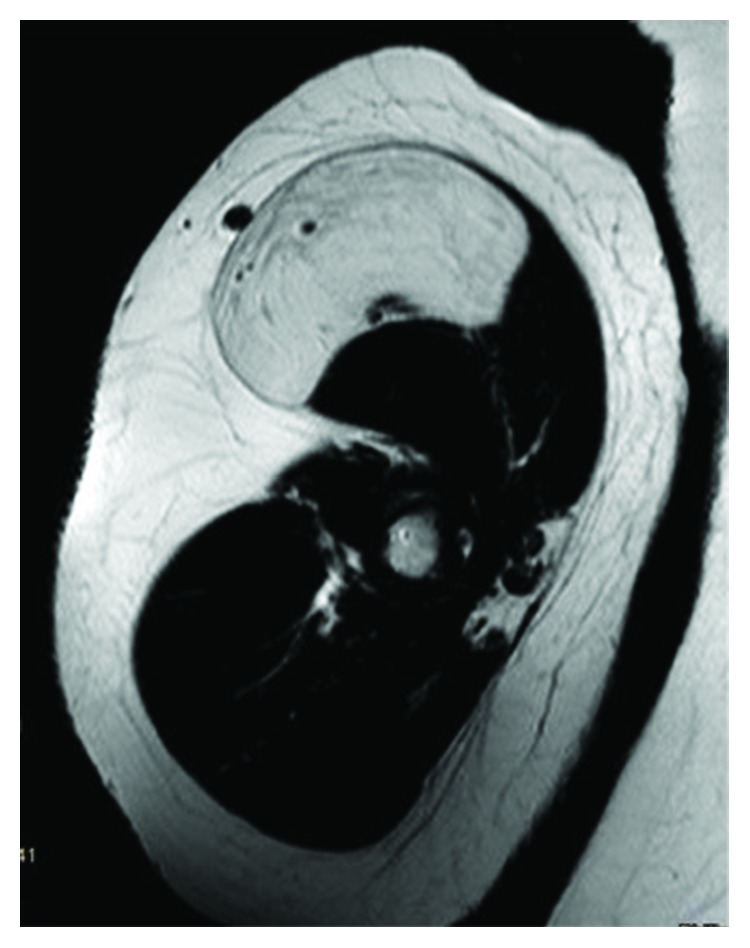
Axial MRI image, contrast-enhanced, T1w.
